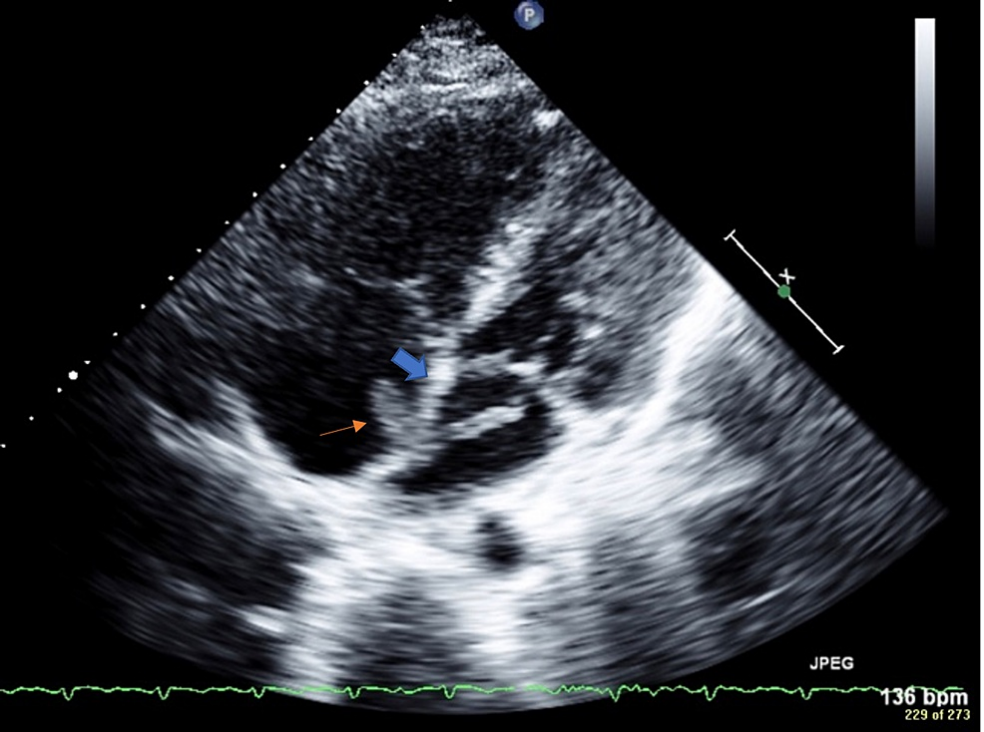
Two-dimensional transthoracic echocardiogram with color flow and Doppler ultrasonography. Two-dimensional transthoracic echocardiogram showing a thrombus (orange arrow) in transit lodged across the interatrial septum (thick blue arrow). One lobe is present in the right atrium, measuring 1.4 cm × 0.6 cm, and one lobe is in the left atrium, measuring more than 1 cm in length.
